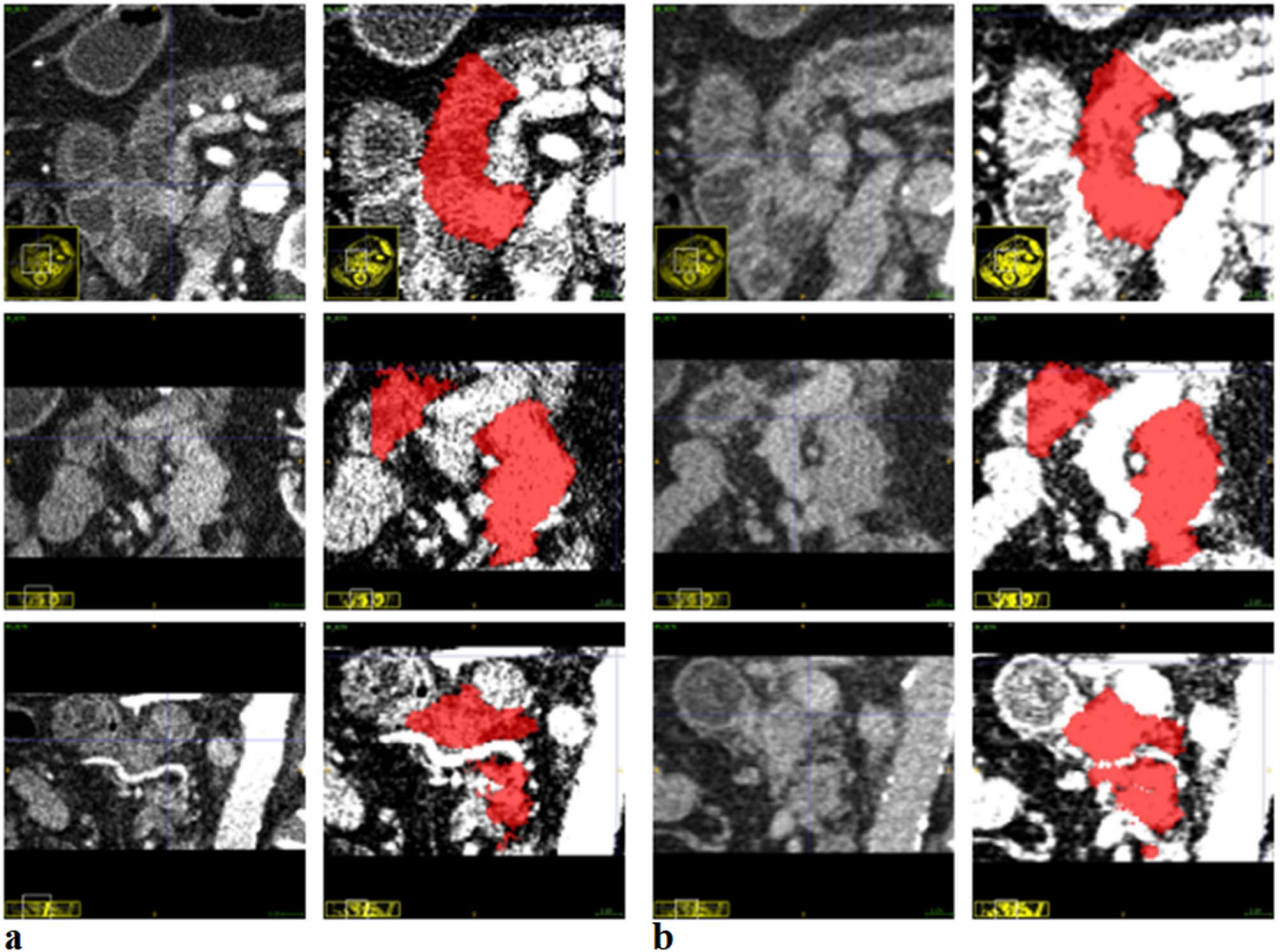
Example segmentation of pancreatic head carcinoma and adjacent pancreatic head parenchyma. (a) Arterial phase; (b) Venous phase. The axial CT series is presented in the top row, the sagittal series in the middle, and the coronal series at the bottom. The segmentation is drawn in red.
